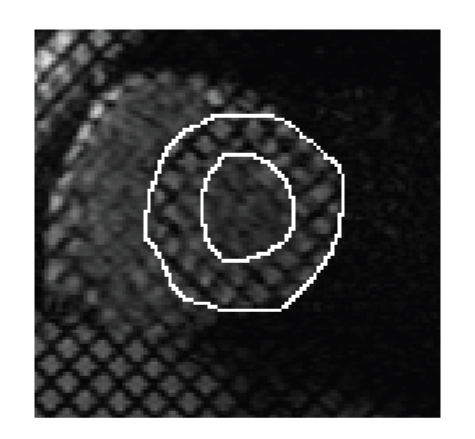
Manual detection of epicardial (external circle) and endocardial (internal circle) boundaries of the Left Ventricle (LV) on a Short-Axis-Tagged MRI acquisition.
